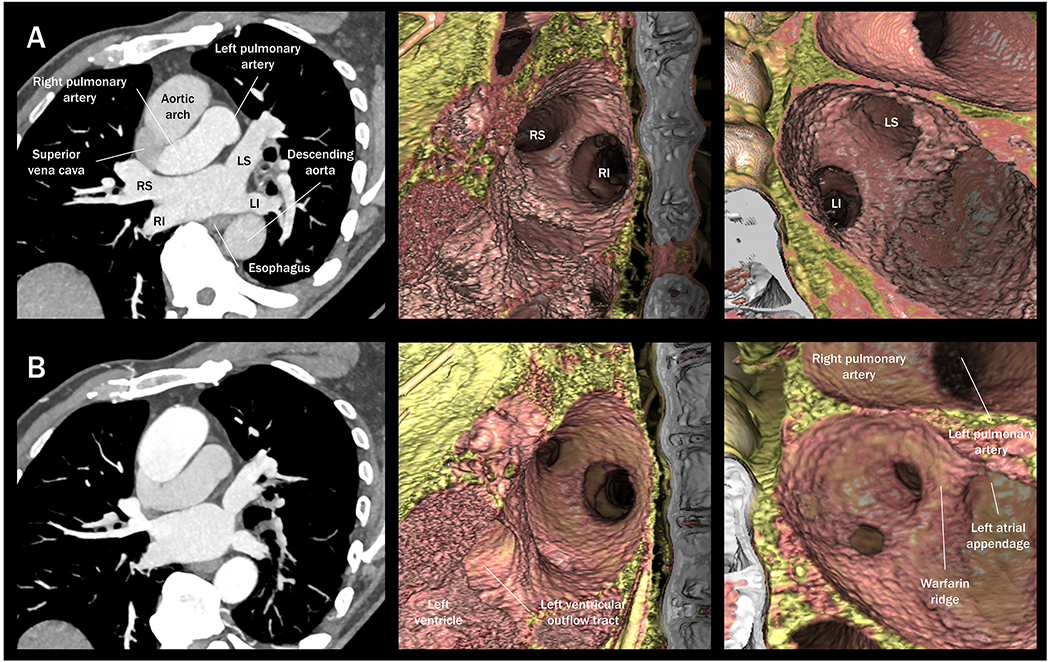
Pulmonary vein stenosis after balloon cryoablation. Cardiac computed tomography images (slab multiplanar reconstruction) before (A) and 8 months after (B) pulmonary vein isolation using a cryoablation balloon reveals moderate stenosis of the right superior, left superior, and left inferior pulmonary vein ostia. Middle panel images (left lateral view) and right panel images (right anterior oblique and caudal view) are volume-rendered virtual dissection images. LI: Left inferior pulmonary vein; LS: left superior pulmonary vein; RI: right inferior pulmonary vein; RS: right superior pulmonary vein.
